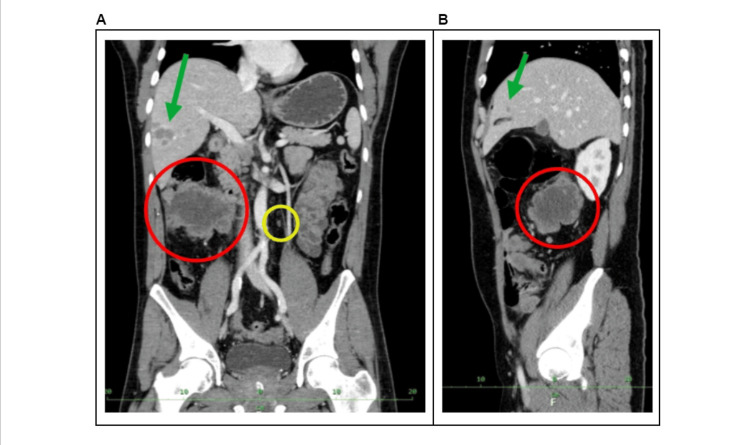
Staging CT examination (venous phase) (A) Anteroposterior reconstruction with the largest tumorous mass situated in the area of the ascending colon (red circle), paraaortal malignant lymph node (yellow circle), and liver metastasis (green arrow). (B) Lateral reconstruction of the abdomen with liver metastasis (green arrow) and tumorous mass in the area of the ascending colon (red circle).
